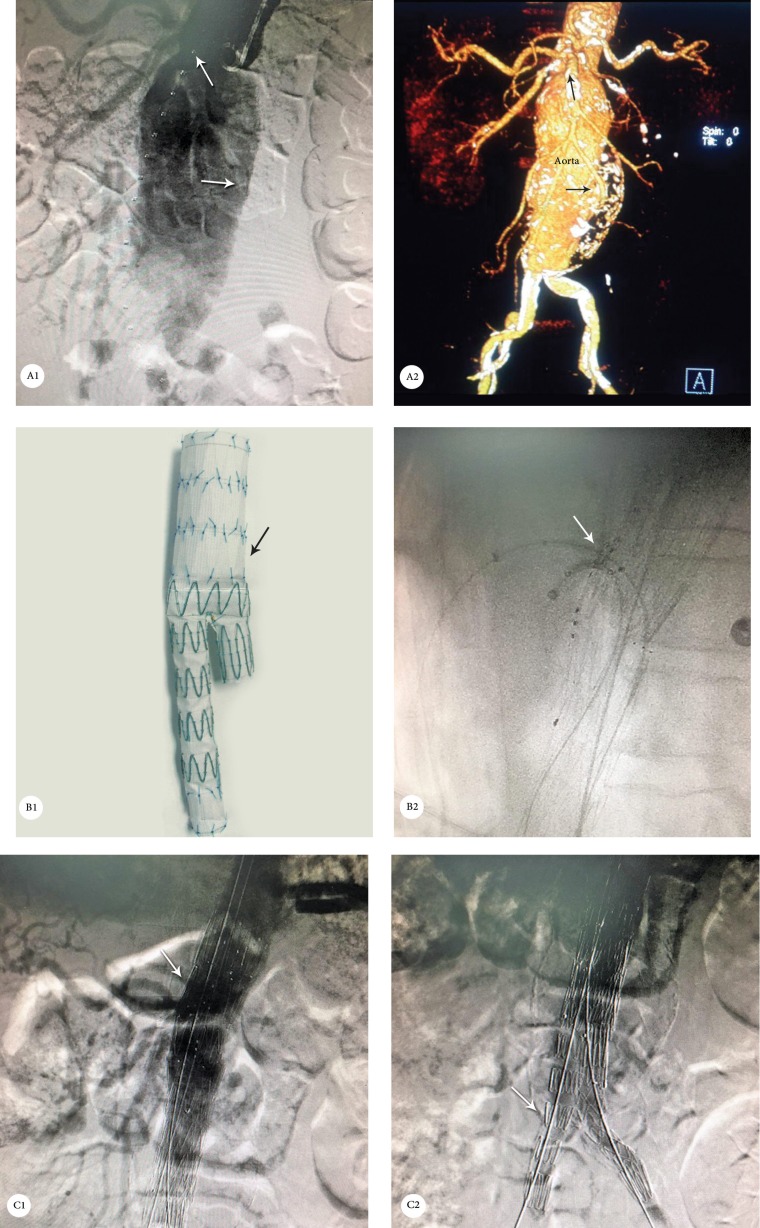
A1 & A2: Abdominal computed tomography angiography, showing a juxtarenal aortic aneurysm (arrows); B1: Main body of the abdominal aortic stent graft; B2: Fluoroscopy, showing the cannulation of the holes of the custom-made fenestrated stent graft; C1: Digital subtraction angiography following fenestrated endovascular aortic aneurysm repair, showing the successful exclusion of the abdominal aorta aneurysm (arrow); C2: Digital subtraction angiography following fenestrated endovascular aortic aneurysm repair, showing patent iliac artery stent grafts (arrow).
